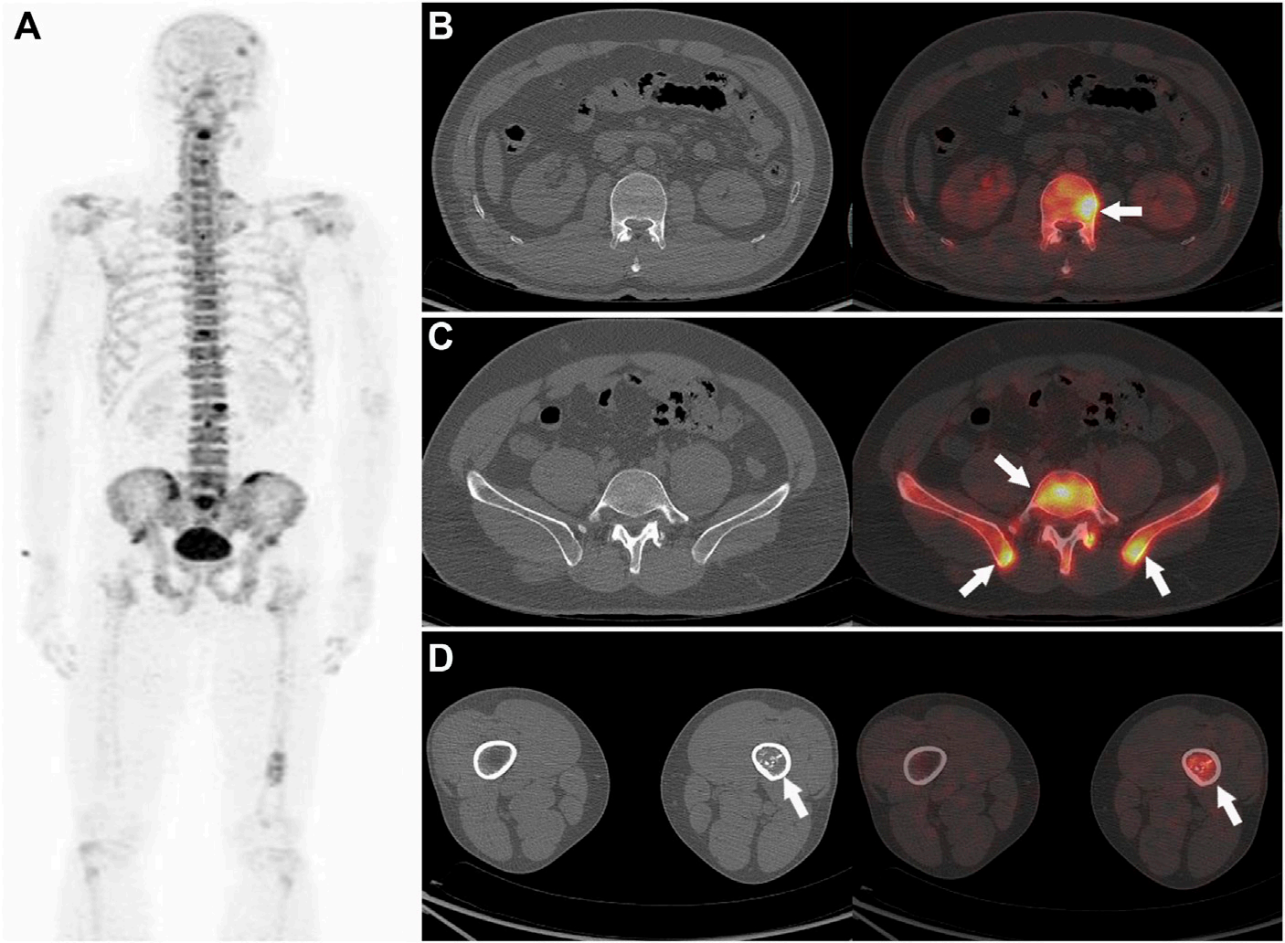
Nasopharyngeal carcinoma (T3M3M1) in a 42-year-old male patient. The PET MIP (A) shows multiple ^18^F-NaF uptakes in the skull, spine, pelvis, and the left tibia. Multiple abnormal ^18^F-NaF uptakes in the spine (B), arrow and pelvis (C), arrow, no abnormal morphology changes can be observed in the corresponding CT, were diagnosed as bone metastases (correctly according to the reference standard). A mild abnormal ^18^F-NaF uptake in the left tibia (D, arrow), and a heterogeneous increase in density in the corresponding CT (D), which were diagnosed as abnormal bone fibre proliferation disease (correctly according to the reference standard).
